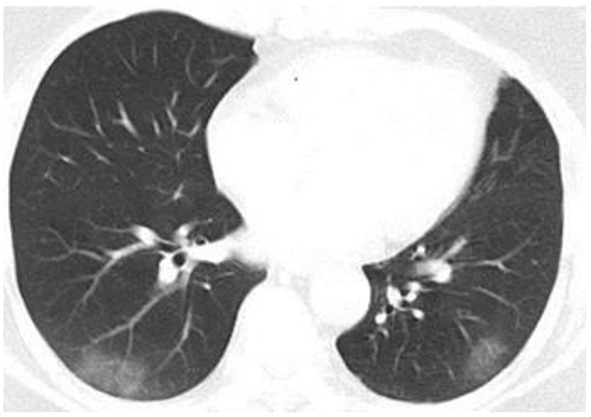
Chest CT image of a COVID-19 patient with hyposmia. Axis chest CT scan showed bilateral patchy ground-glass opacities consistent with typical moderate COVID-19.
